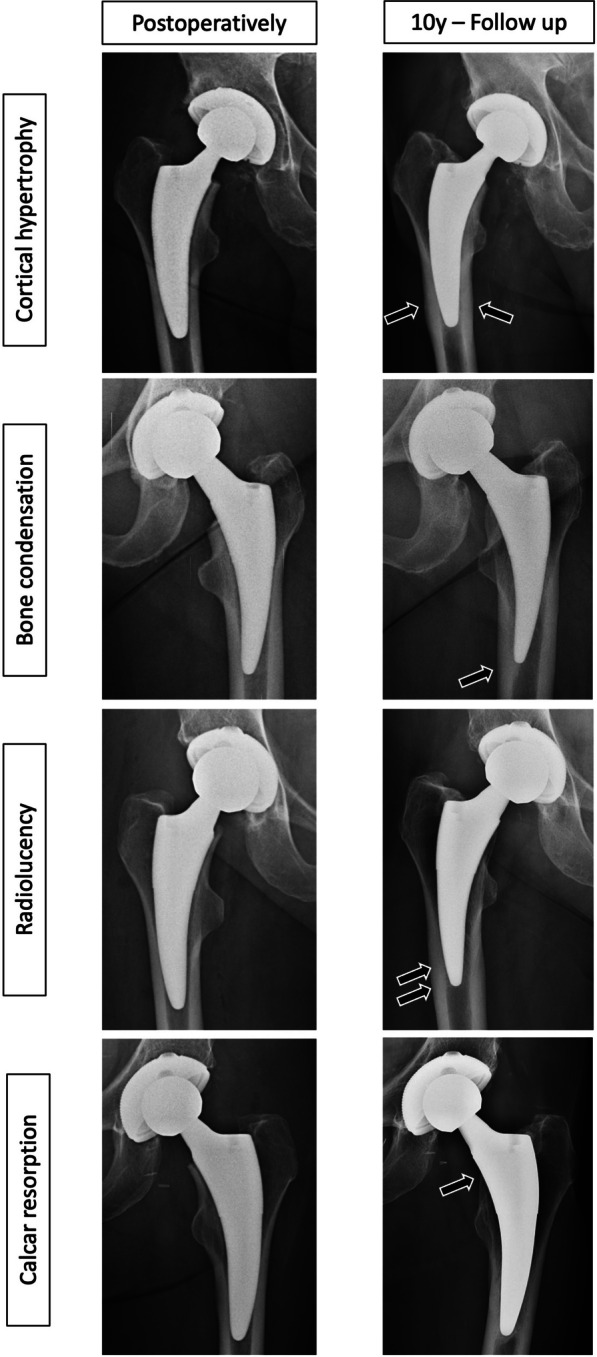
Radiological outcome parameters on ap radiographs immediately postoperatively and after 10 years: Radiolucent lines, calcar resorption, bone condensation and cortical hypertrophy
